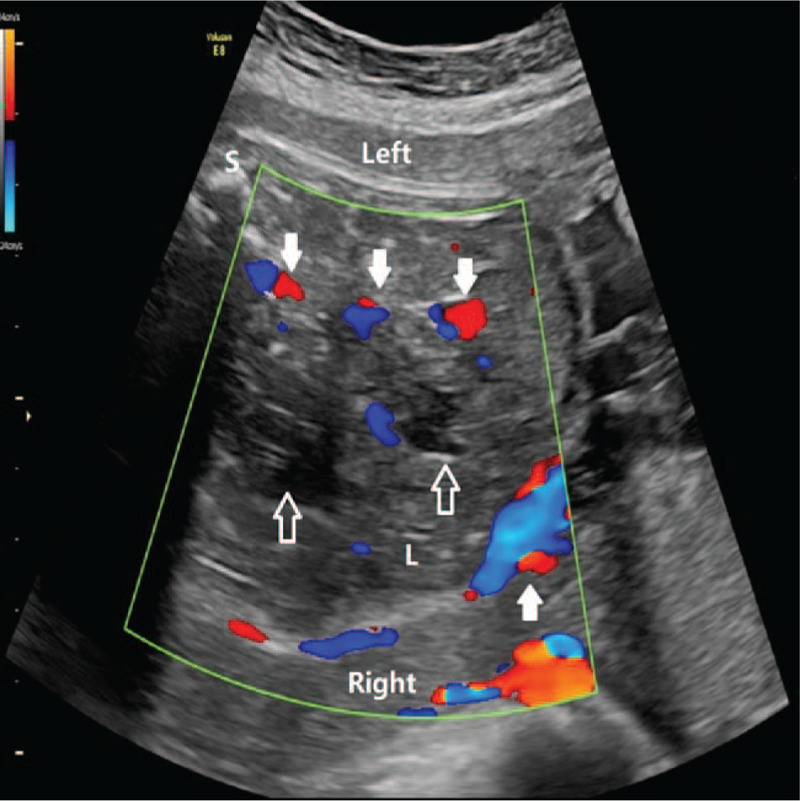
Ultrasonic features in B-mode and color Doppler. A heterogeneous solid mass located in the right quadrant of the fetal abdominal cavity, with close relationship to the liver. The lesion seemed to have ill-defined margin, irregular shape, partial capsule and multiple cystic cavities inside (hollow arrow). The mass appeared to be highly vascularized (solid arrow). (s: spine, L: liver, Left: the left side; Right: the right side).
